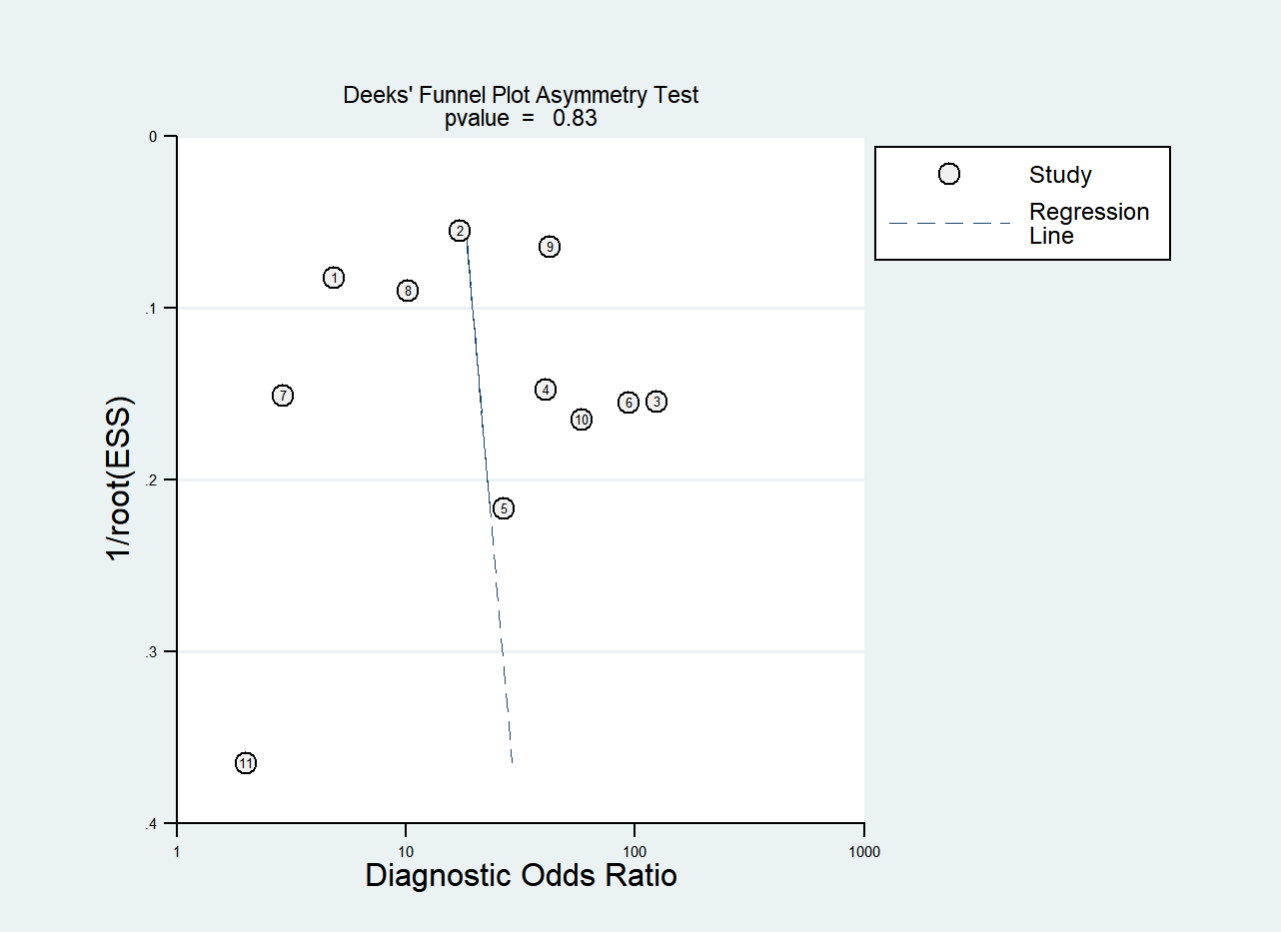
Deek’s funnel plot for radiomics studies based on CT images in distinguishing between lung adenocarcinoma and lung squamous cell carcinoma.
